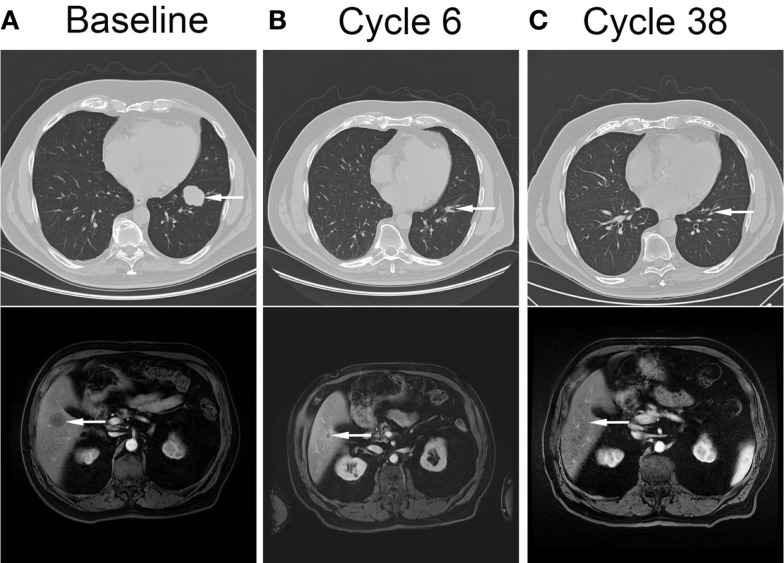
Complete resolution of metastatic disease in response to cytotoxic therapy and veliparib. Computed tomography images showing pulmonary (top panel, arrow) and liver (bottom panel, arrow) metastases at baseline (A), after completion of cytotoxic therapy and veliparib (B), and after 38 cycles of veliparib maintenance therapy (C).
